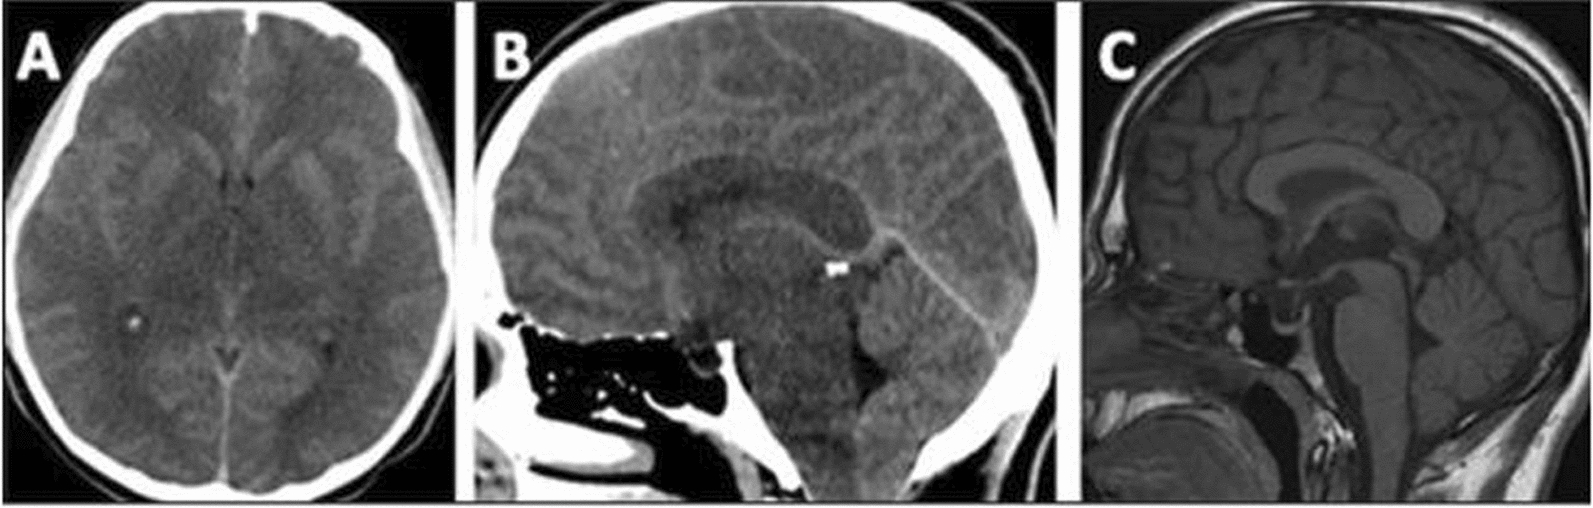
Brain imaging of patient 1 Head-CT at the initial presentation shows diffuse brain edema (A and B), causing cerebellar tonsillar herniation (B). Sagittal T1 from the following MRI brain shows improved edema and resolution of tonsillar herniation (C)
